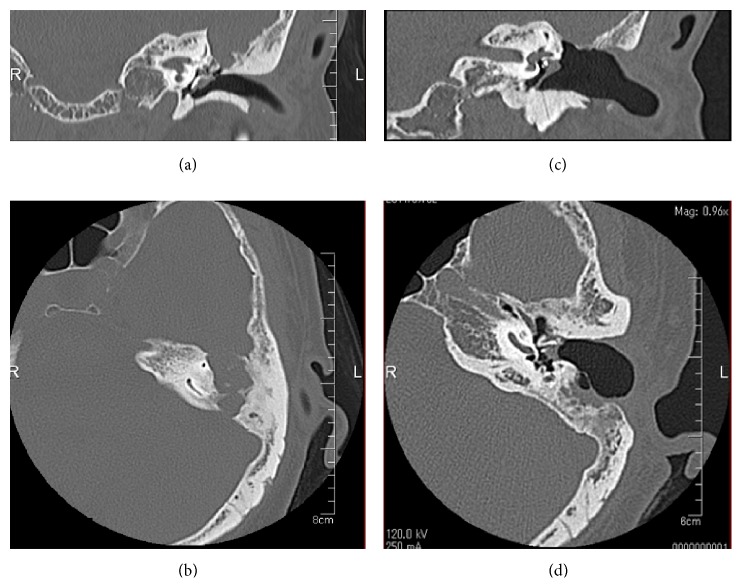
(a) Coronal CT of the temporal bone showing bony destruction of the middle cranial fossa. (b) Axial CT showing bony destruction of the middle and posterior cranial fossae. (c, d) Postoperative CT at 3 years after the operation shows no recurrence of cholesterol granuloma.
